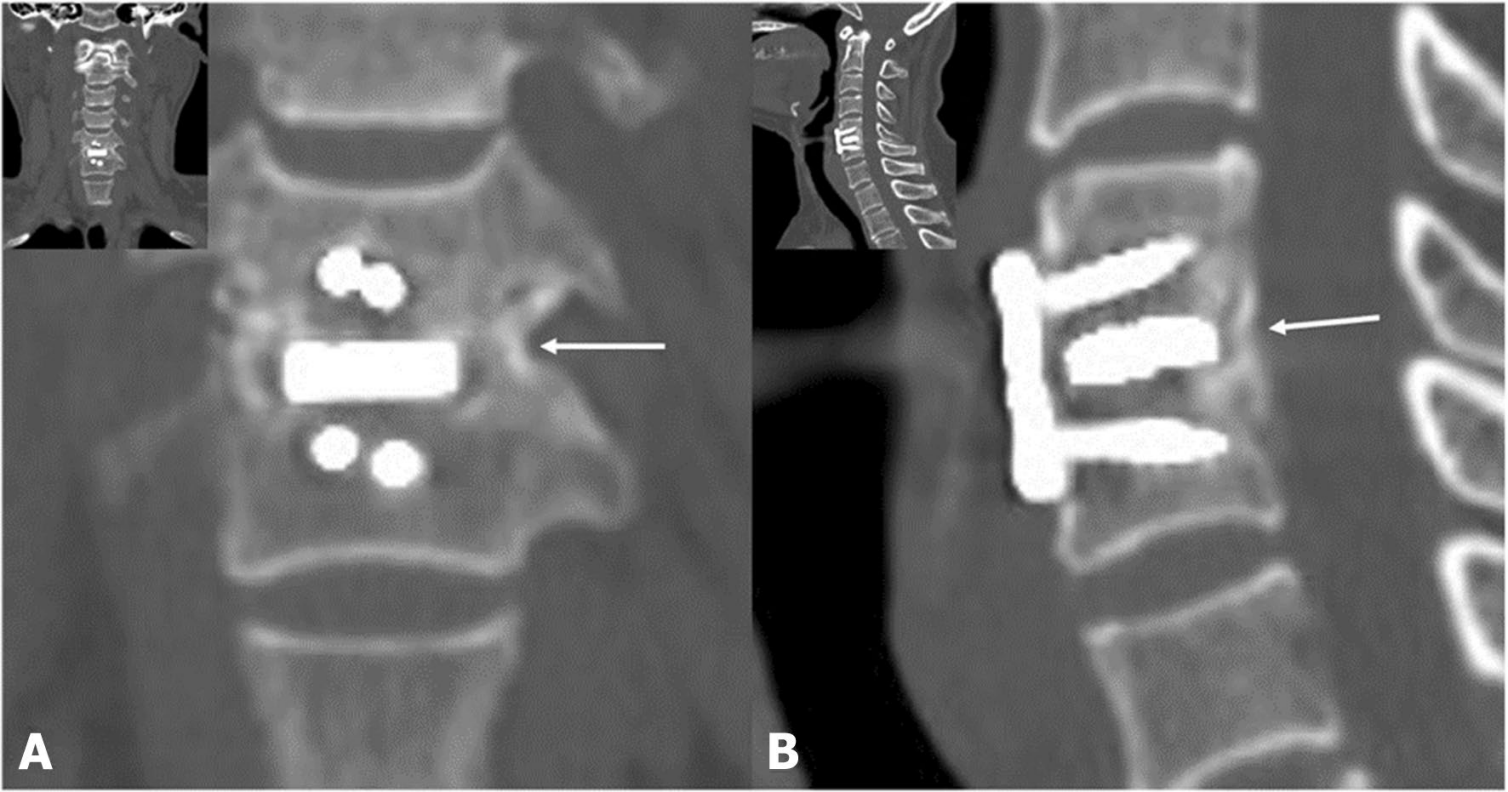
12 months postoperative CT image. Bone bridge formation (white arrows) was assessed in coronal (A) and sagittal (B).
